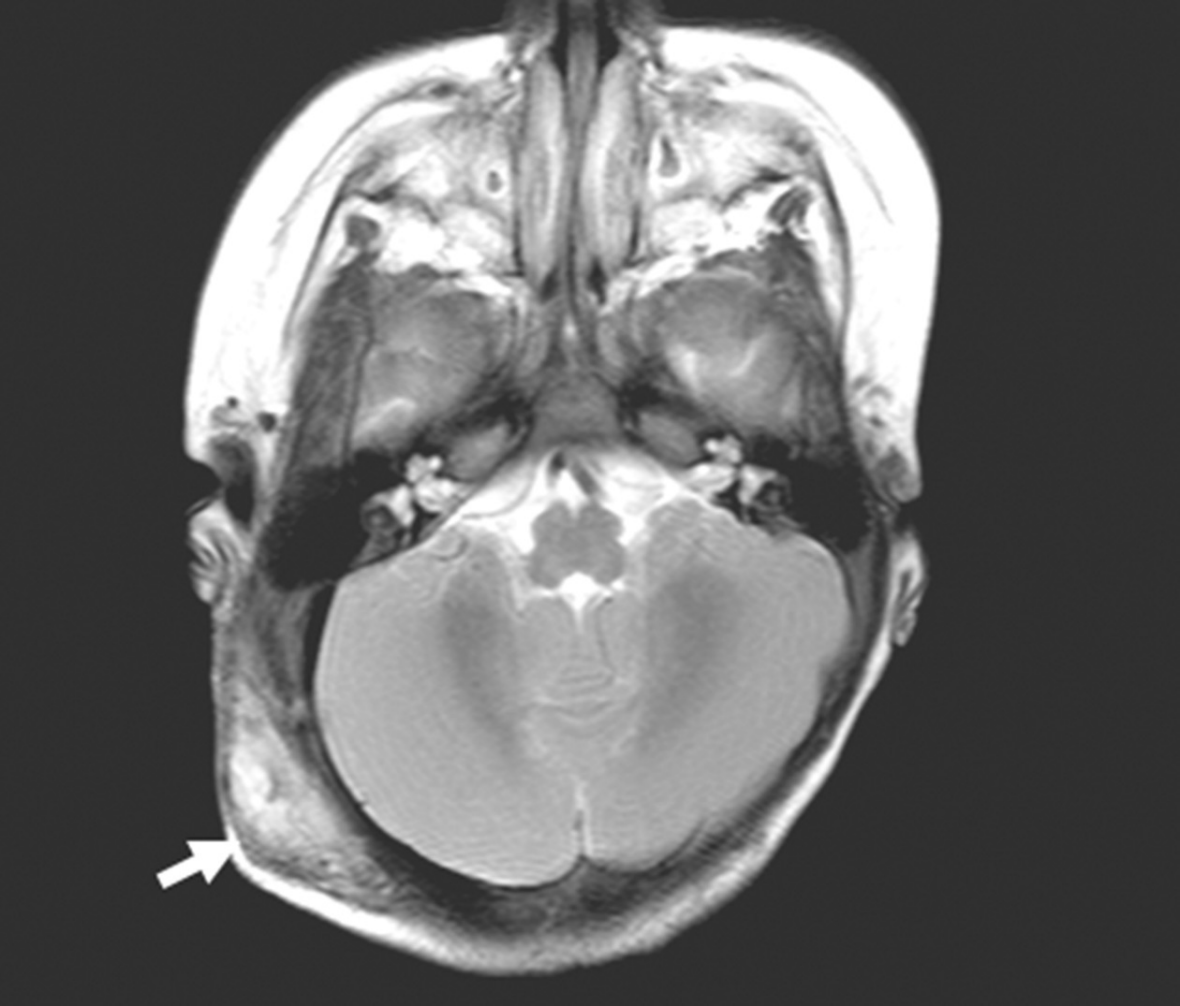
The *T*_2_ weighted MRI obtained 6 months after embolization shows a significant decrease in the tumour size (arrow).
